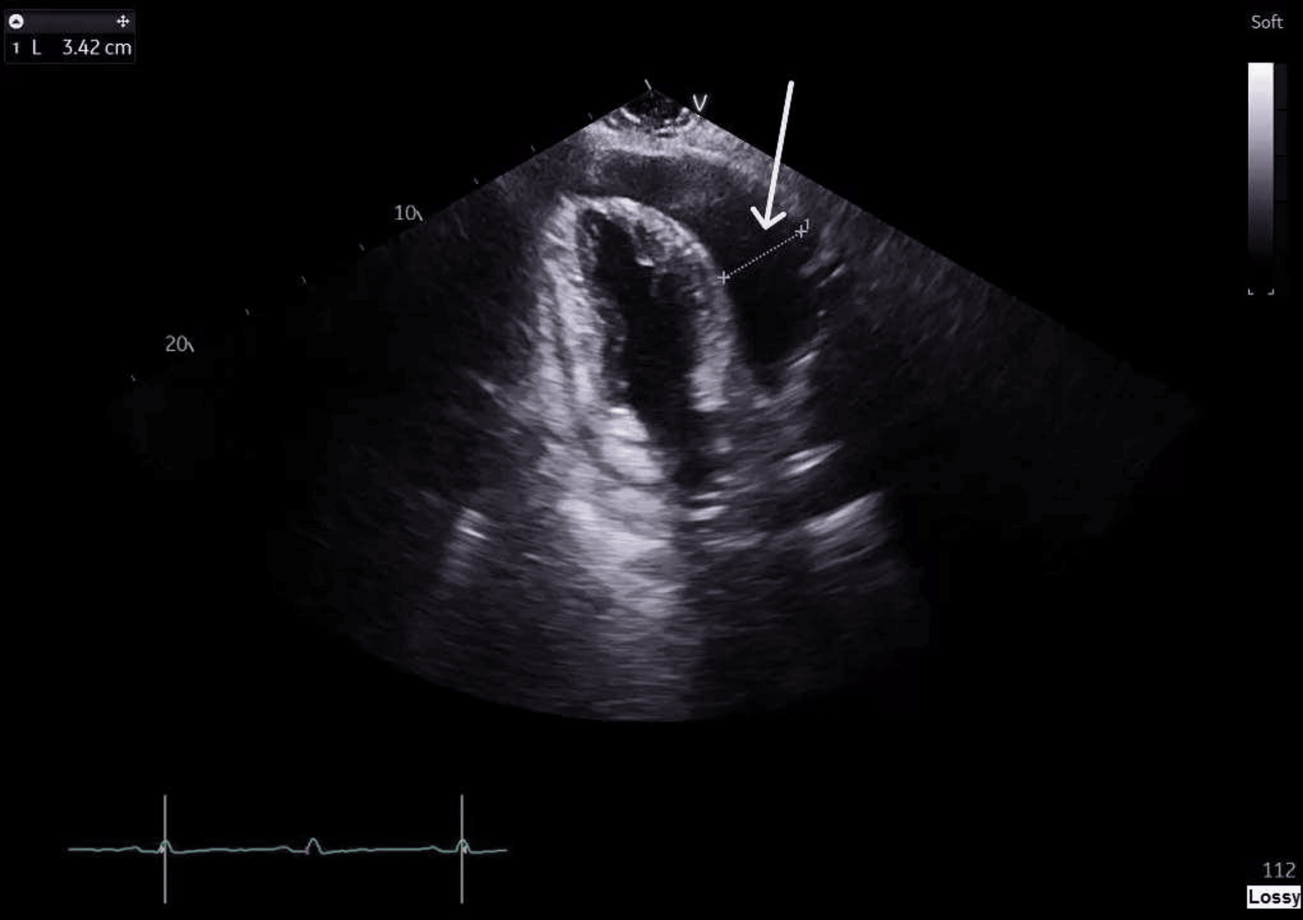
Transthoracic echocardiography, apical two-chamber view, showing the anterior dimension of the pericardial effusion measuring 3.42 cm The white arrow indicates the effusion at its greatest dimension.
